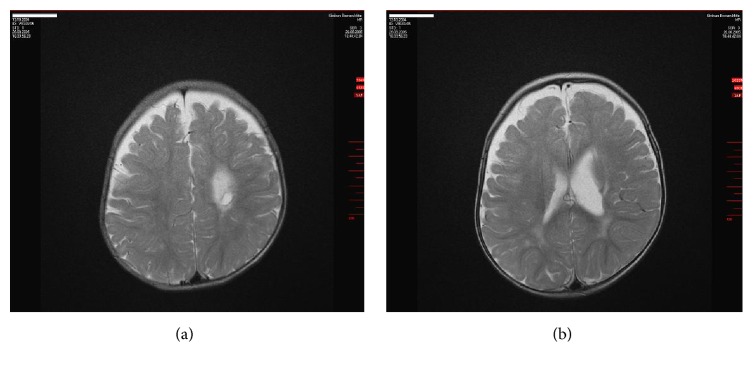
MRI of the brain (M.H.) at 10 months of age (2005). Left ischemic stroke in the central and paraventricular white matter resulting in a porencephalic cyst and enlarged lateral ventricle (1.5-Tesla Avanto, head coil array, T2w TSE sequence).
